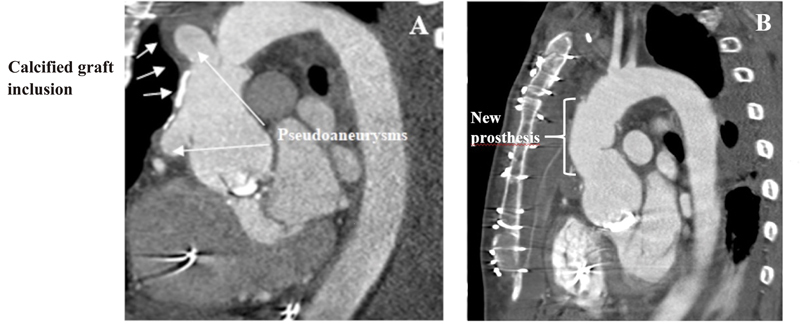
Preoperative computed tomography (CT) scan with (
